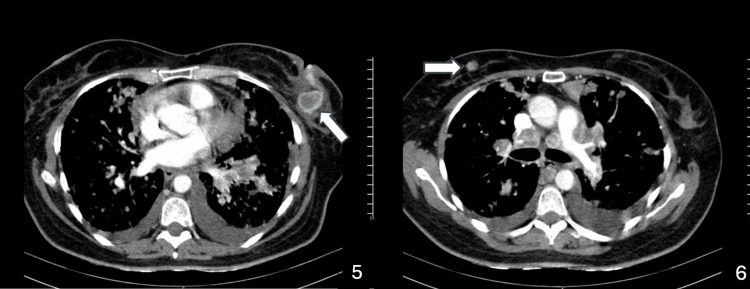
Axial CT scans demonstrating the metastatic spread of renal cell carcinoma to both breasts (arrows), with associated mediastinal lymphadenopathy and bilateral pleural effusions.
